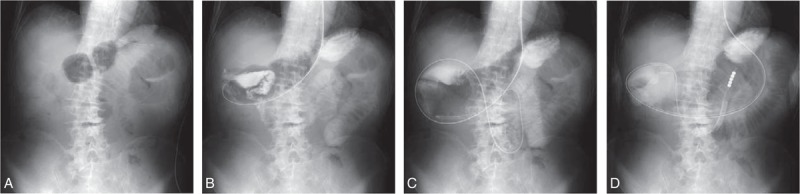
Radiographs of NEWSt. (A) A short nasogastric tube (NGT) is placed in the stomach. (B) A Dennis guidewire (1.32 mm, 500 cm long; Covidien, Tokyo, Japan) is inserted via NGT. (C) The guidewire is advanced beyond the duodenojejunal flexure. (D) After NGT is withdrawn, a hydrophilic long tube (16 Fr, 300 cm; Argyle Super Dennis Tube; Covidien, Tokyo, Japan) is inserted along the guidewire, which is withdrawn. NEWSt = nonendoscopic over-the-wire method via short nasogastric tube.
